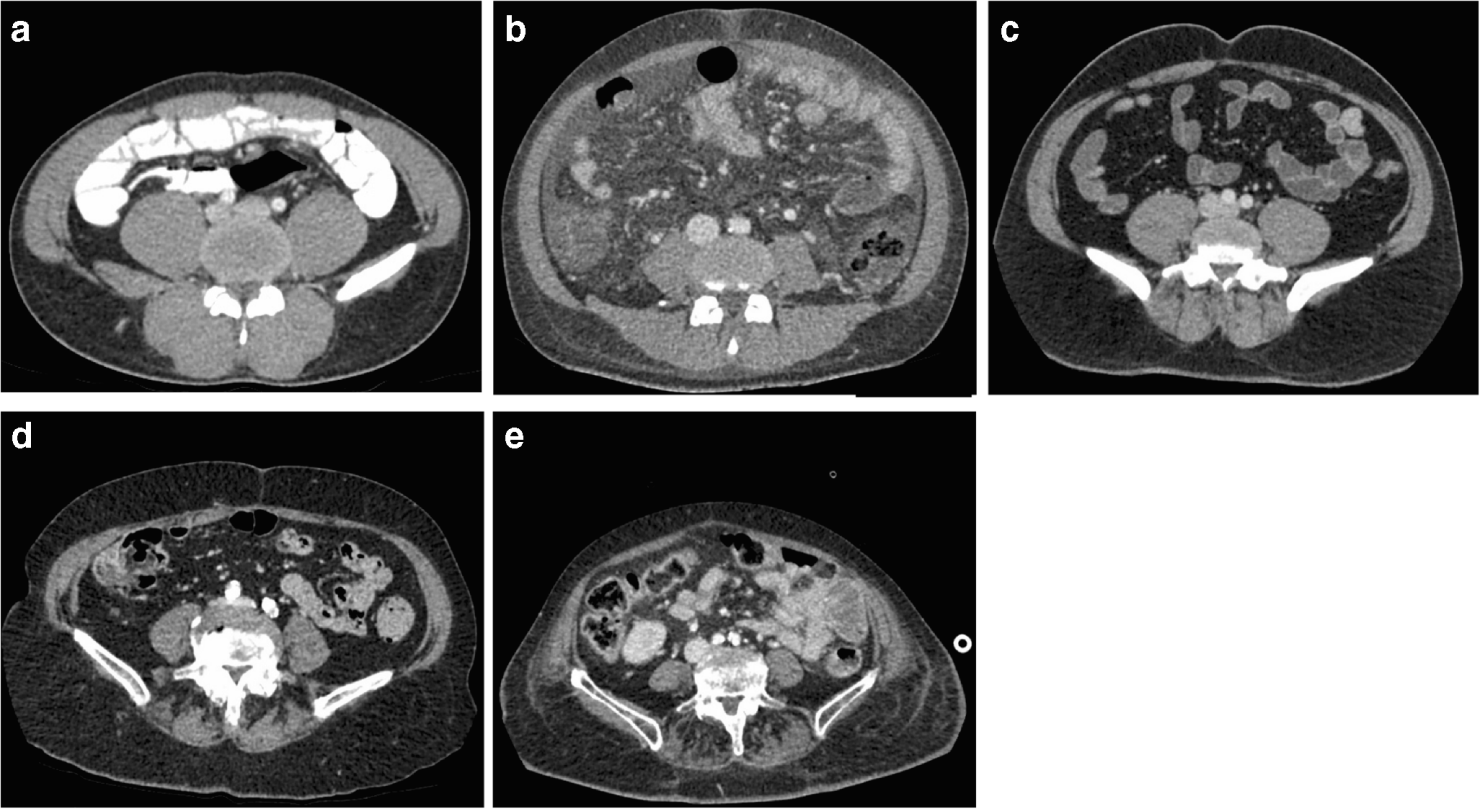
CT scans showing different Goutallier gradings of the paravertebral muscles at level L4/5. a. Grade 0: no fatty infiltration. b. Grade 1: some fatty streaks. c. Grade 2: less than 50% fatty infiltration. d. Grade 3: 50% infiltration. e. Grade 4: more than 50% fatty infiltration
